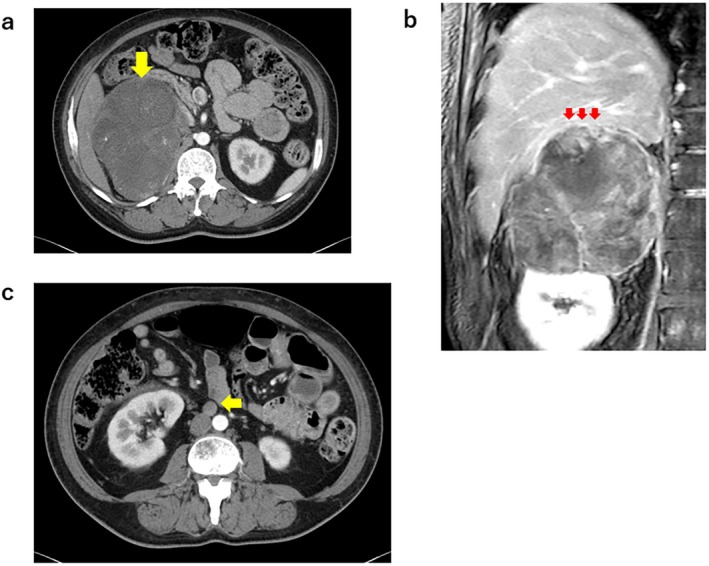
Imaging findings of a right adrenal tumor. (a) Enhanced CT finding of a right adrenal tumor. (b) Enhanced MR finding of a right adrenal tumor. Arrow indicates a suspected liver invasion. (c) Enhanced CT finding of a right adrenal tumor and a paracaval lymph node metastasis (indicated by arrow).
